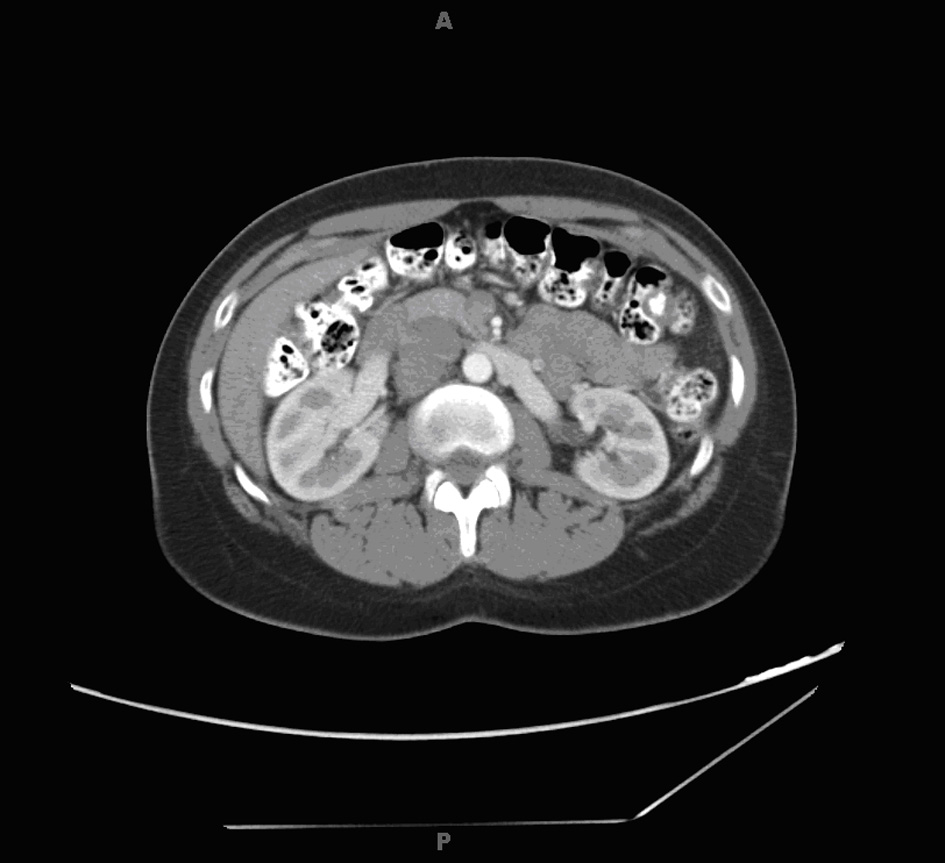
Abdominal computed tomography scan showing right paracaval mass.
